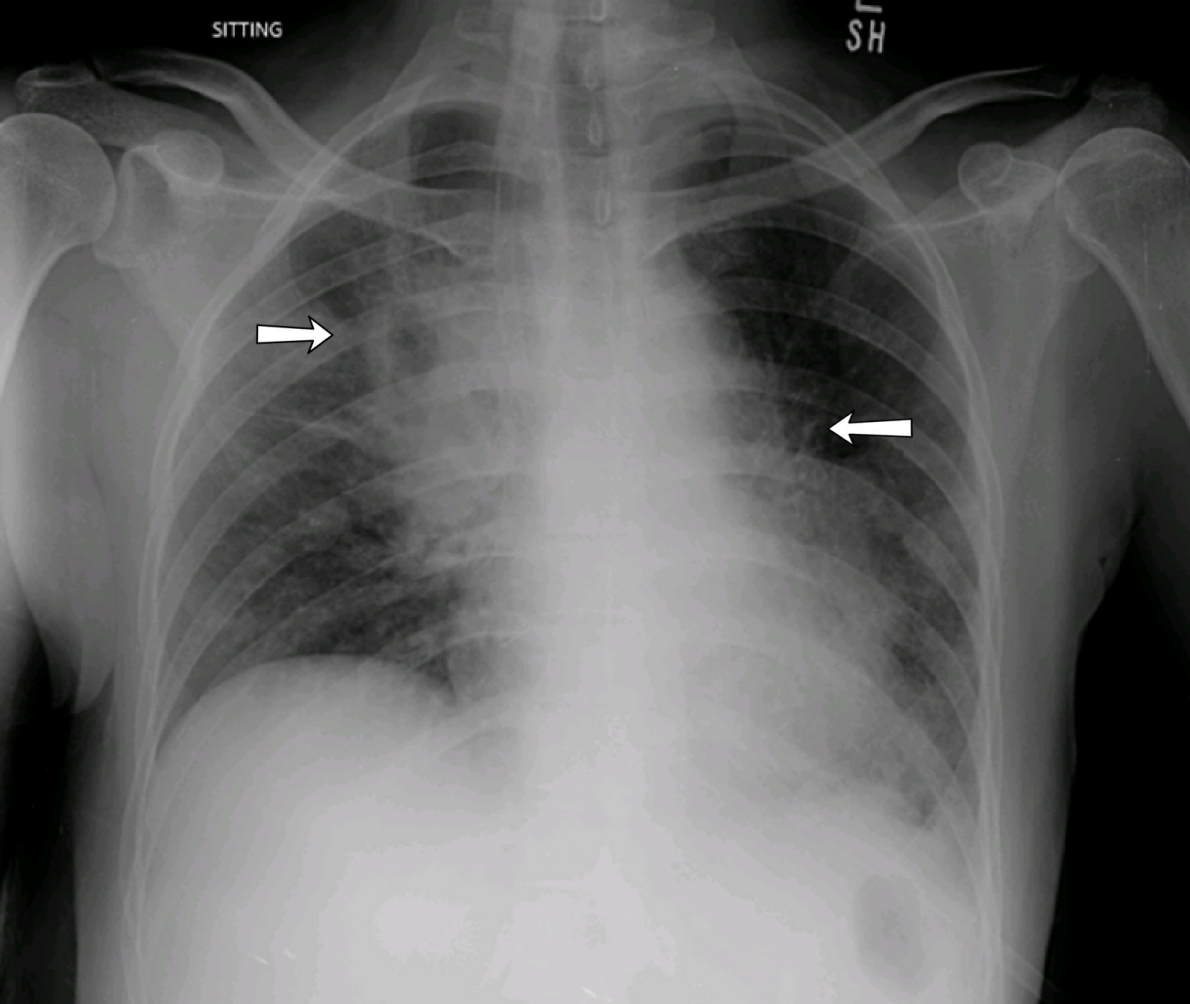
Image showing the chest X-ray. Non-homogenous shadowing with mild volume loss and opacification over the right upper heart border (white arrow).
